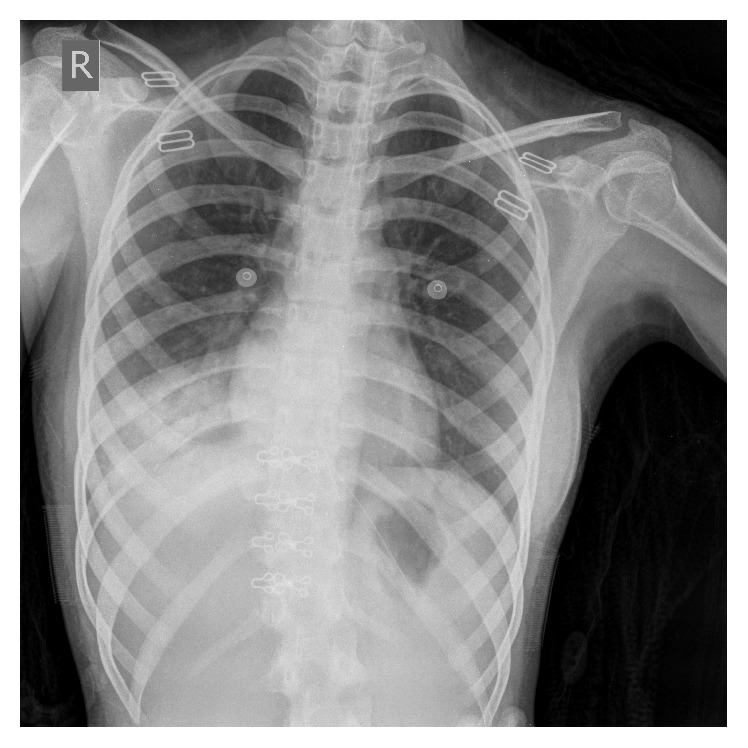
PA chest X-ray demonstrating right lower lobe opacity.
